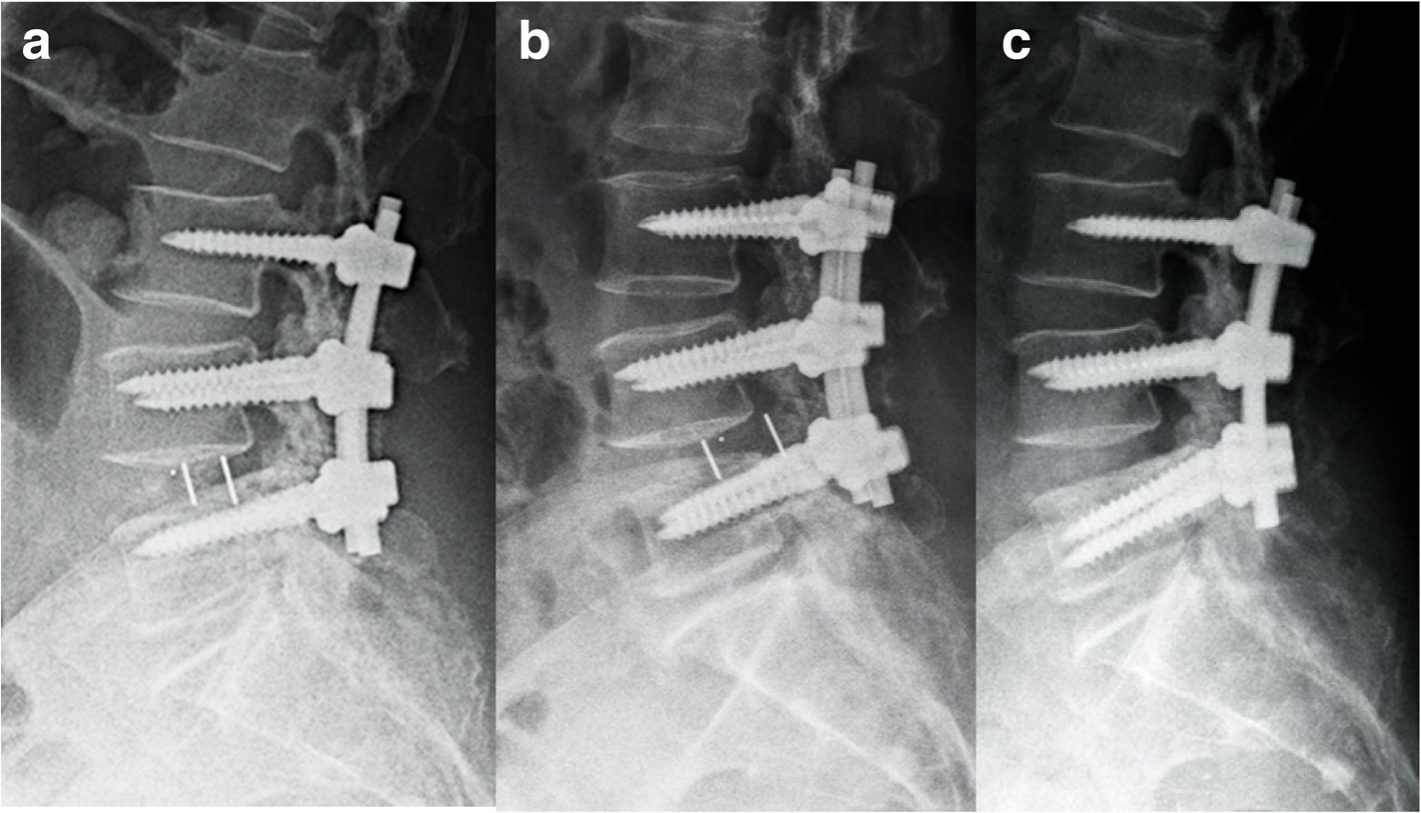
A 73-year-old female was diagnosed with degenerative spinal disease and was treated with L4/5 TLIF and L3-L5 bilateral posterior instrumentation. a Lateral radiograph 3 days postoperatively. b PCM at L4/5 13 days postoperatively. c Lateral radiograph obtained 2 days after revision surgery. The migrated cage was removed and the loosened right L5 pedicle screw was revised
